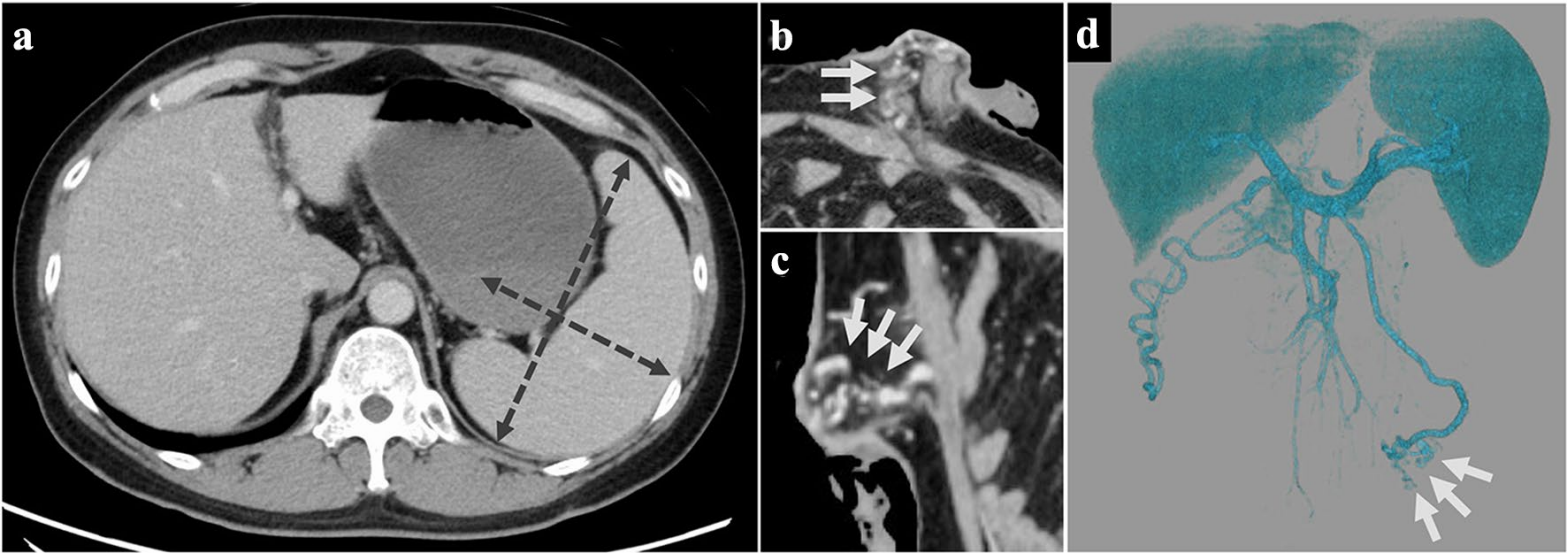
Contrast-enhanced CT findings at admission. a Severe splenomegaly 17 × 7 cm (dotted arrow). No liver metastases were found. b–d Varicose veins around the stoma (arrow) perfused into the IMV (b axial, c sagittal, d 3D angiography)
